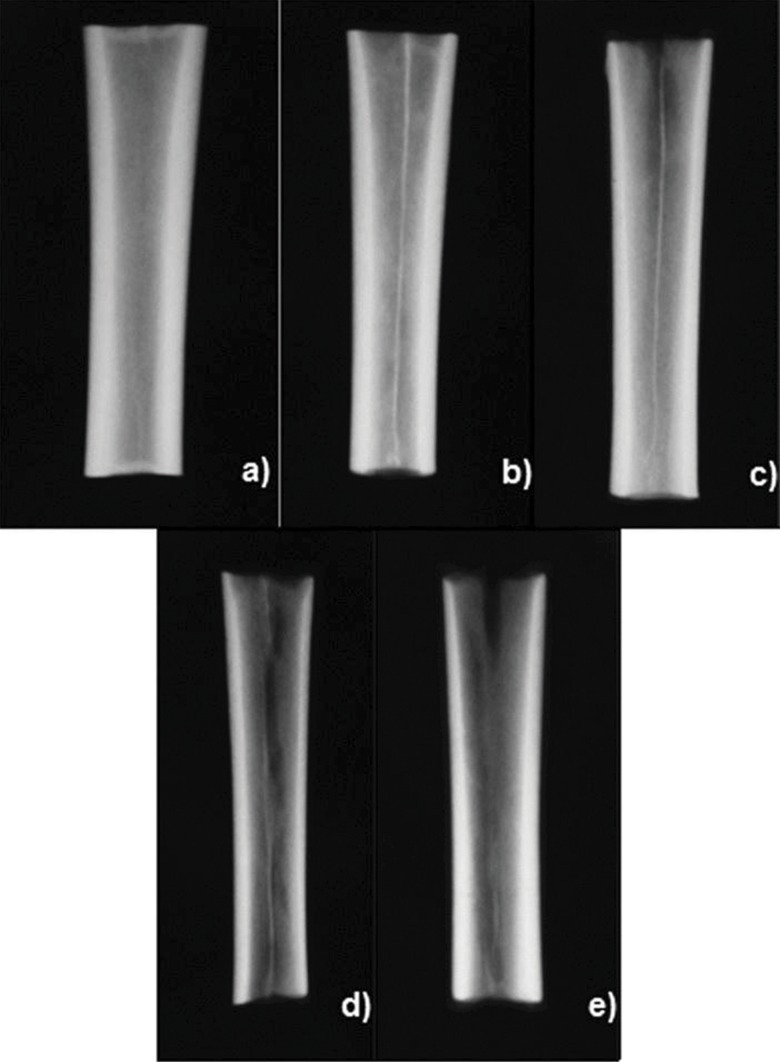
Radiographic appearance of the bone segments after the demineralization process: before demineralization (a) and after 6 (b), 12 (c), 24 (d) and 36 (e) hours. Differences are not apparent, except for some thinning of the cortex and rarefaction of the medullary cancellous bone.
